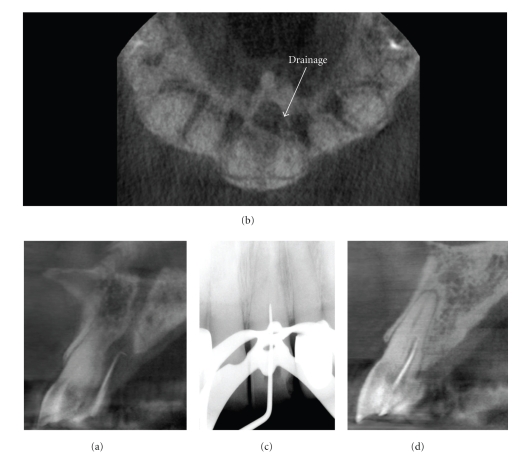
A 79-year-old male was referred for endodontic treatment of the maxillary left central incisor after a palatal sinus tract was noted. CBCT imaging was initially performed with a gutta percha cone marker inserted into the sinus tract to determine the source of the infection. Sagittal images (a) demonstrated that the lesion terminated at the periapex of the maxillary left central incisor after coursing through the incisive canal whereas drainage was visible on the axial image (b). Perioperatively, only the mesiodistal direction could be determined on conventional intraoral periapical radiography (c) and treatment suspended when the explorer reached 17 mm because of the danger of perforation in the facial or palatal direction. Subsequent cross-sectional perioperative CBCT imaging (d) with an intracanal gutta percha marker indicated that the initial access preparation was directed palatally. Correction of the access facially resulted in gaining access to the apical terminus; treatment was completed without complication.
